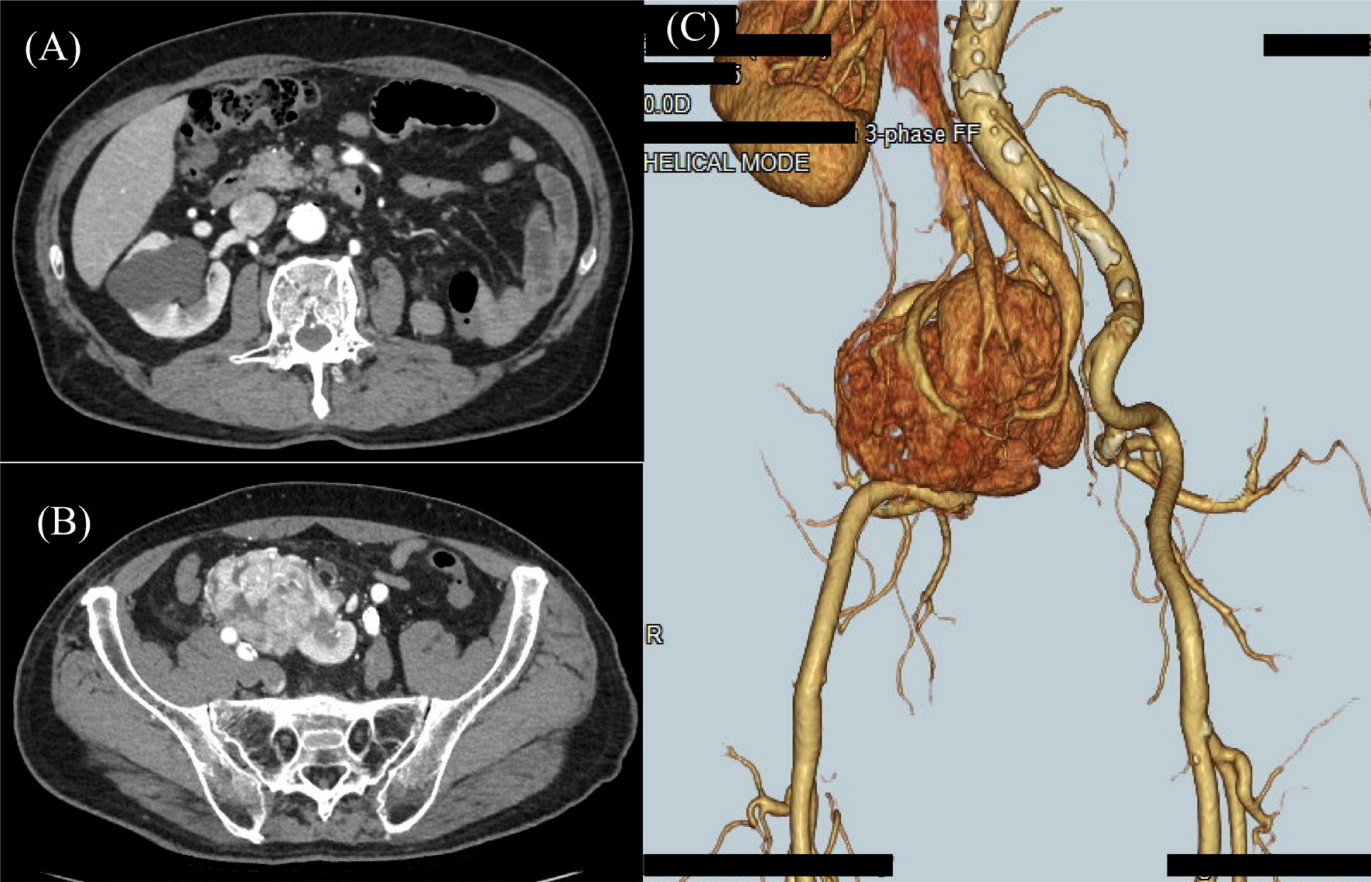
Preoperative CT findings of the pelvic kidney. (A) Axial contrast‐enhanced CT image shows the absence of the kidney in its normal anatomical position. (B) CT shows a 60 mm enhancing mass in the left pelvic kidney, which is located anterior to the sacrum. (C) Three‐dimensional CT shows clearly these findings. CT, computed tomography.
